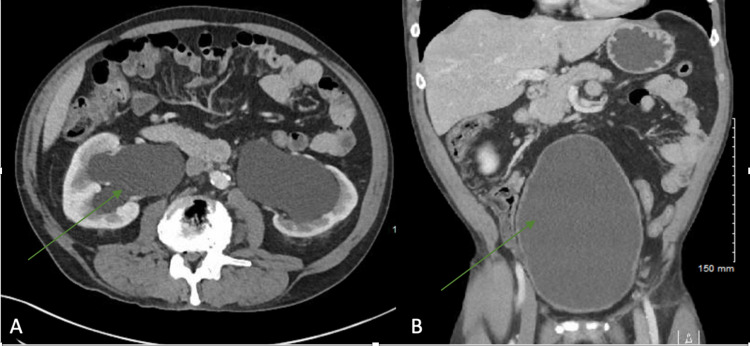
Computed tomography scan of abdomen of (A) axial and (B) coronal sections showing hydroureteronephrosis and distended bladder.
